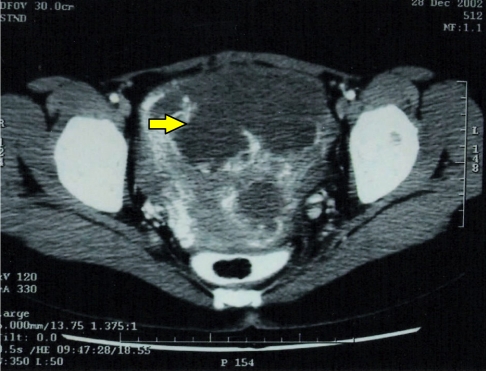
Computed tomography scan of the pelvis revealed a highly vascular enhancing mass (arrow) in the pelvis.
